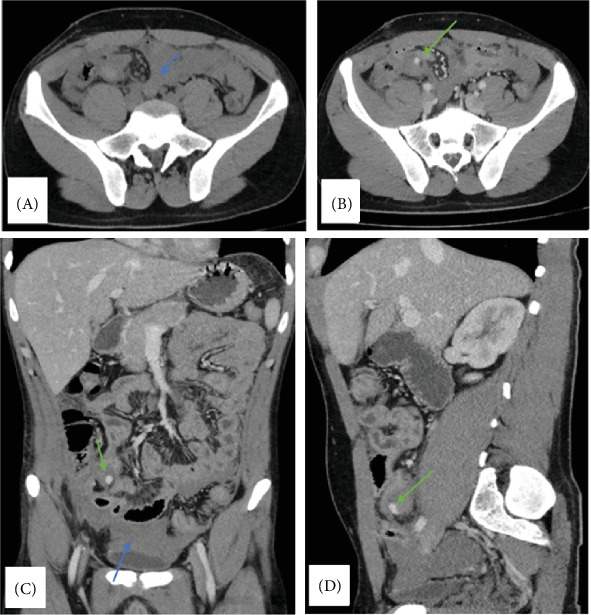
CT abdomen without and with IV contrast-portal phase; in axial (A, B), coronal (C), and sagittal (D) planes, moderate amount of free fluid with high density suggestive of hemoperitoneum with clots formation was noted predominantly at right iliac fossa (blue arrow), in addition, focal hyper dense area was noted on post contrast images at site of removed appendiceal stump raise the possibility of peritoneal bleeding related to appendiceal stump (green arrow).
